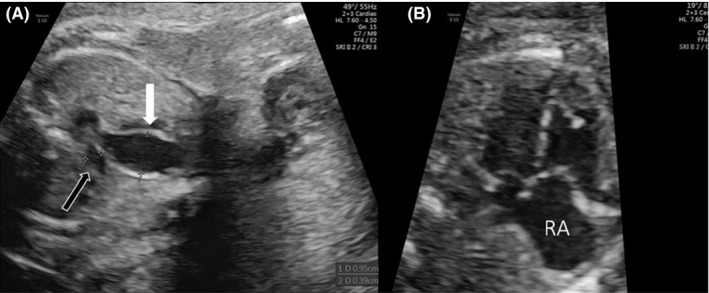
Ultrasound examination at 27 + 5 weeks of gestation showing the severely dilated pulmonary artery of 9.5 mm in size (filled arrow) in comparison with the ascending aorta (continuous arrow) of 3.9 mm in size (A). Four‐chamber view demonstrating the dilated right atrium (RA) at 23 + 3 weeks of gestation (B)
